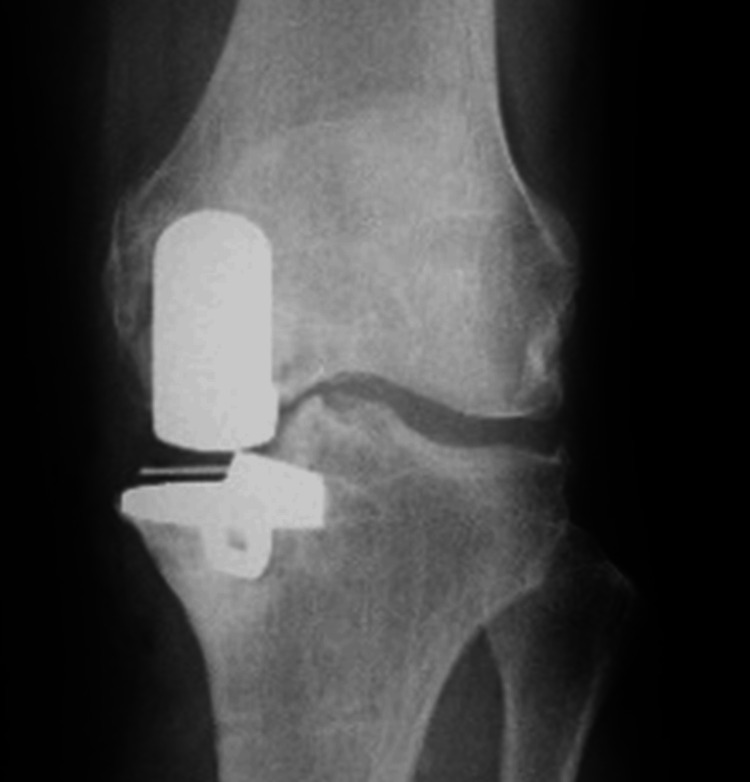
Postoperative radiography after plate removal (AP view)
